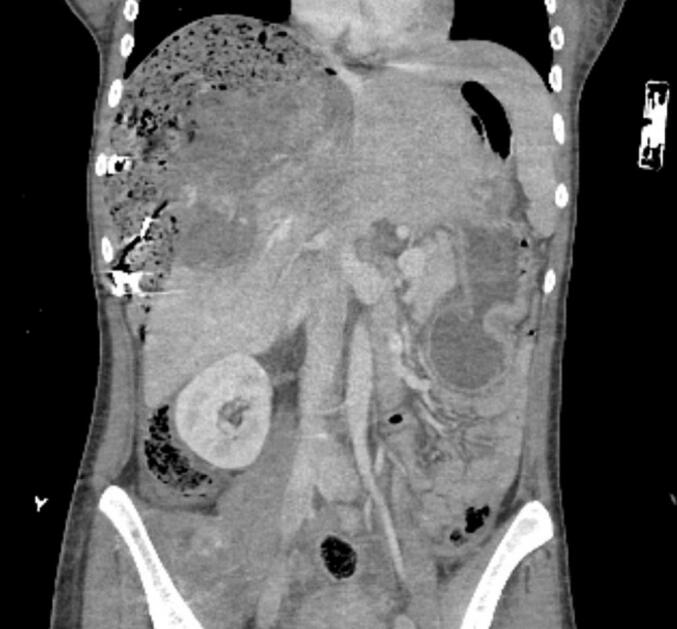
CT scan of the first patient performed after emergency transportation from a different hospital. CT scan demonstrates hepatic metastases and surgical clothes used for abdominal packing to stop hemorrhage from hepatic metastases.
